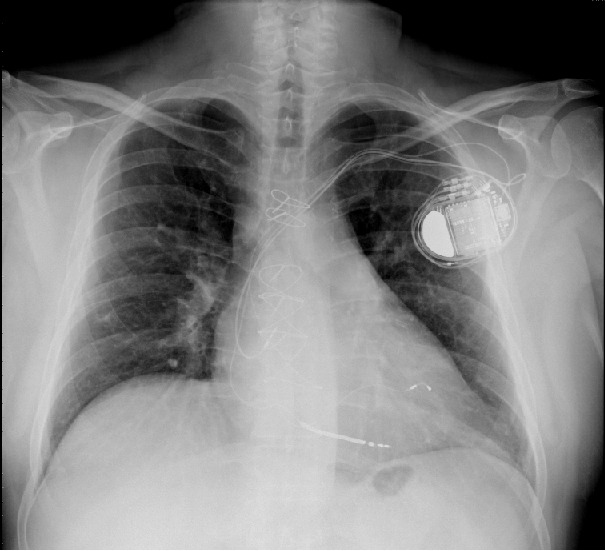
Chest X-ray, postero-anterior view showing a BiotroniK CRT-D with a RA lead, RV high voltage lead, RV pacing/sensing lead and LV lead in the coronary sinus.
